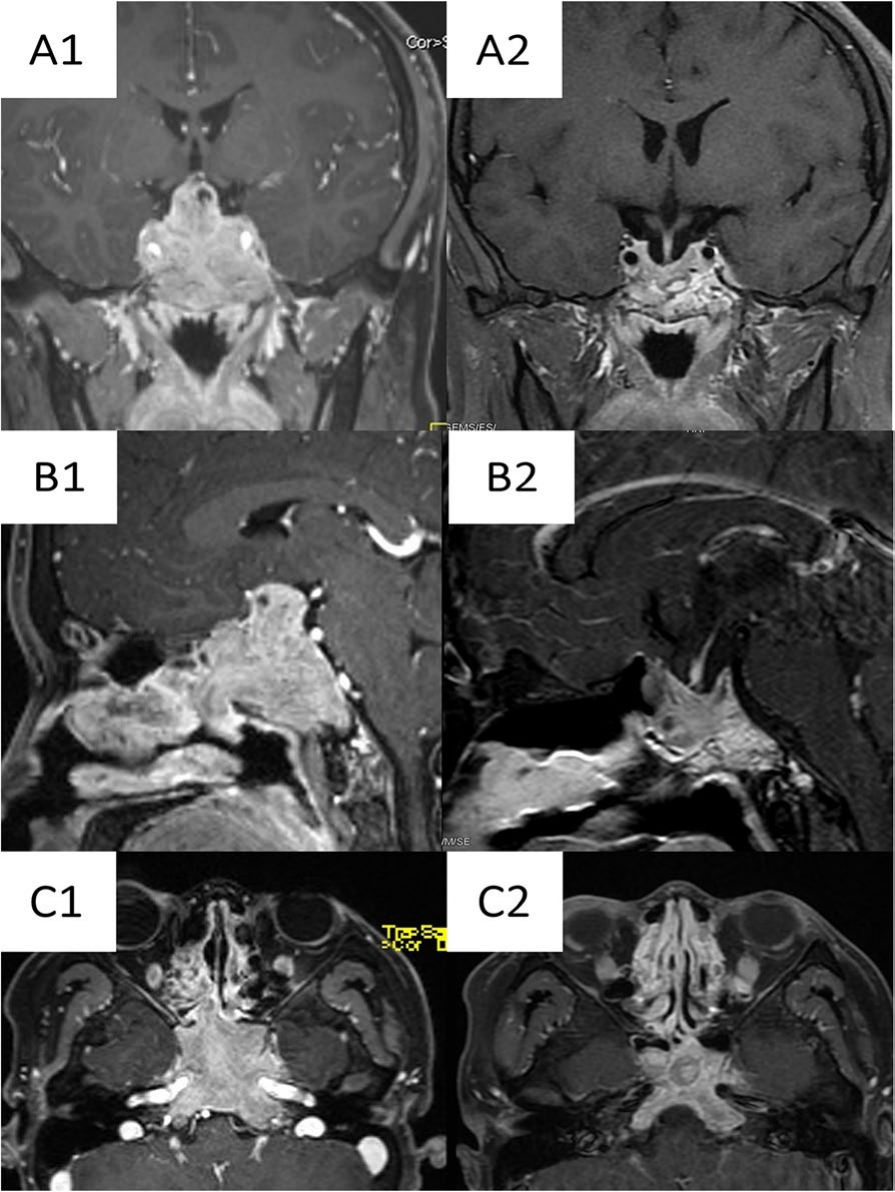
Pituitary enhanced magnetic resonance imaging (MRI) showing tumor shrinkage after treatment with a dopamine agonist in the young patient with a macroprolactinoma. MRI scans obtained before dopamine agonist therapy, showing a large pituitary mass in coronary, sagittal and axial station (A1, B1 and C1, respectively) and after 1 year of treatment, with marked reduction of the mass (A2, B2 and C2, respectively). Before treatment with bromocriptine, an irregular mass about 7.8 cm × 4.6 cm × 5.0 cm was observed on the saddle. It invades the sphenoid and cavernous sinuses, with encasement of the carotid arteries and optic chiasm compression
